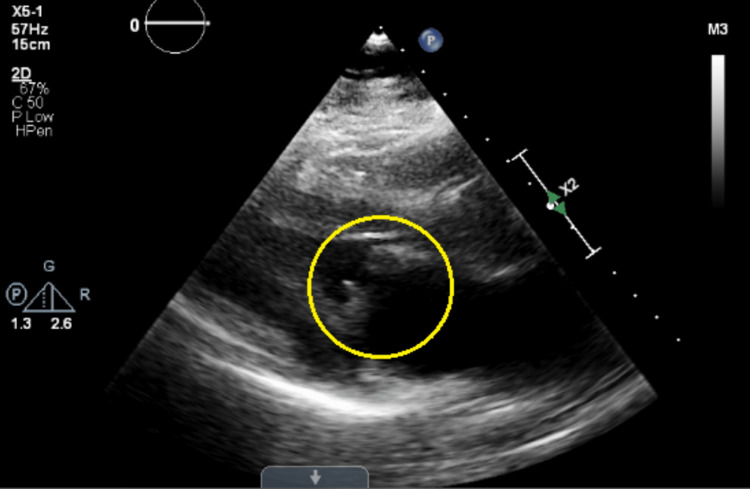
Transthoracic echocardiography showing thickening of mitral valve leaflets (yellow circle)
